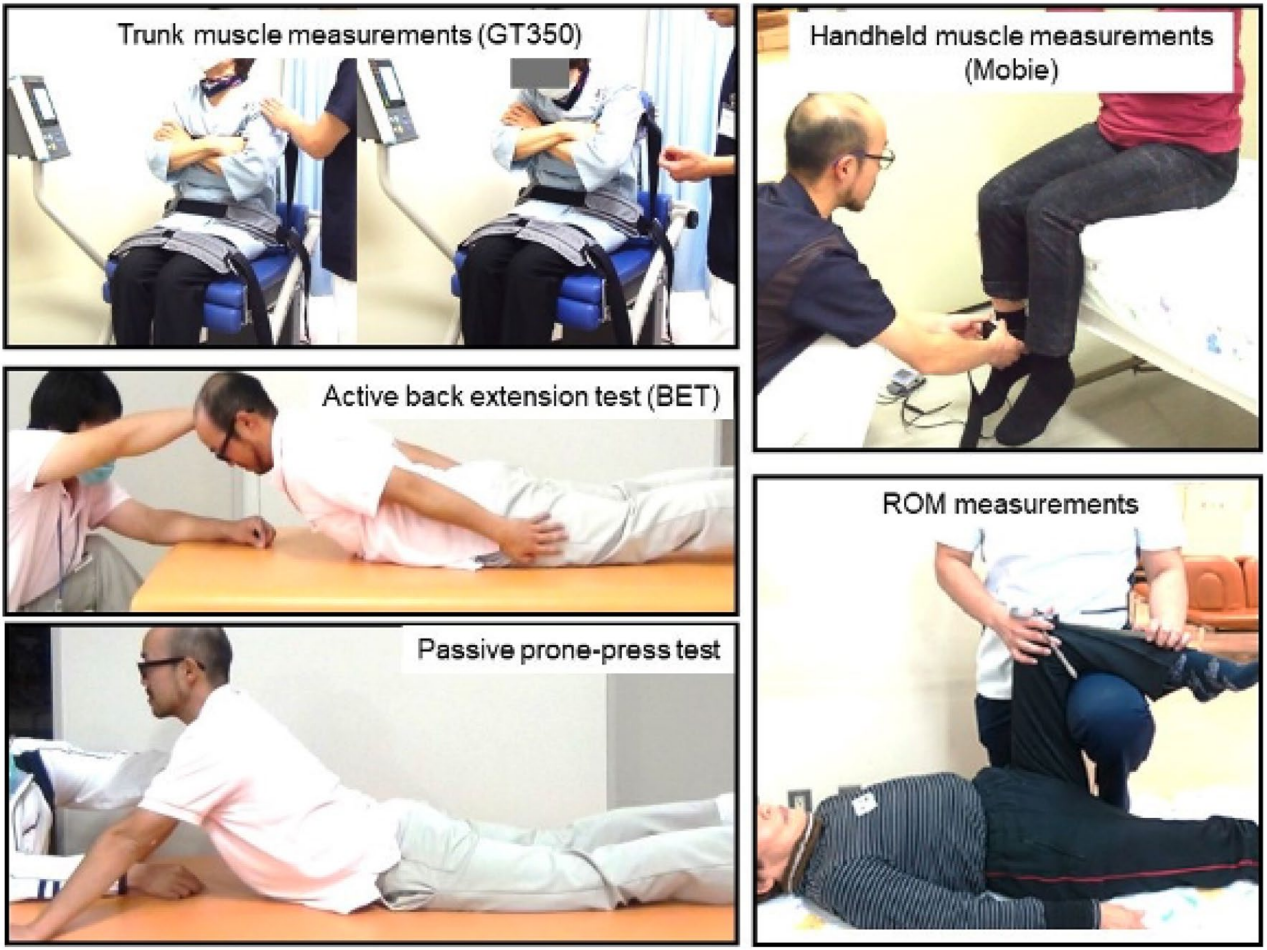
Non-radiographic measurements Trunk flexor and extensor muscle strength measured using isometric device (GT350, OG Giken Co., Japan). Lower extremities muscle strength using isometric device (a handheld dynamometer Mobie of Sakai Med Co.). Active back extension test (BET: distance of sternal notch and floor at active lumbar extension). Passive prone-press test (distance of chin and floor at maximal lumbar extension by push-up force). ROM measurements of lower extremity.
